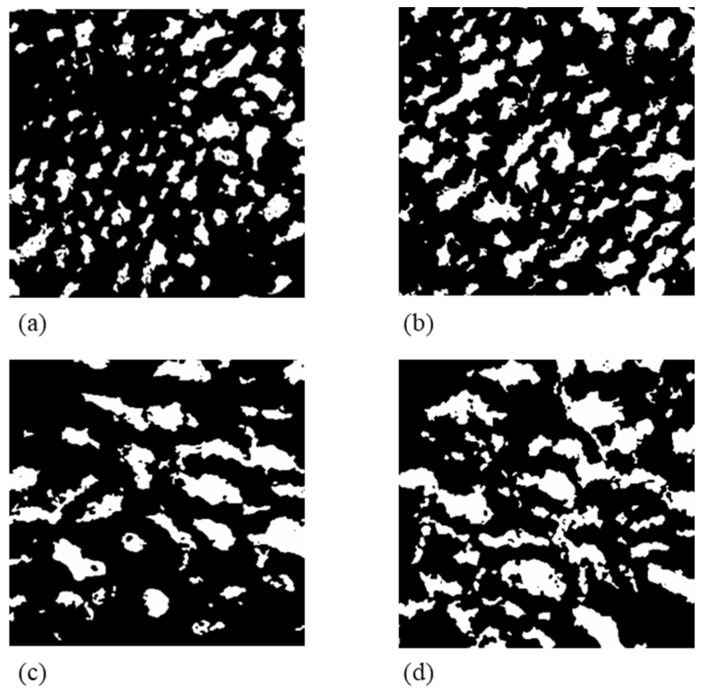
Binary 2D-X-ray images of cutting surface (left) and base (right) of “Golden Delicious” fresh-cut apples samples treated with DoS 1 (a,b) and DoS 4 (c,d) on day 0.
